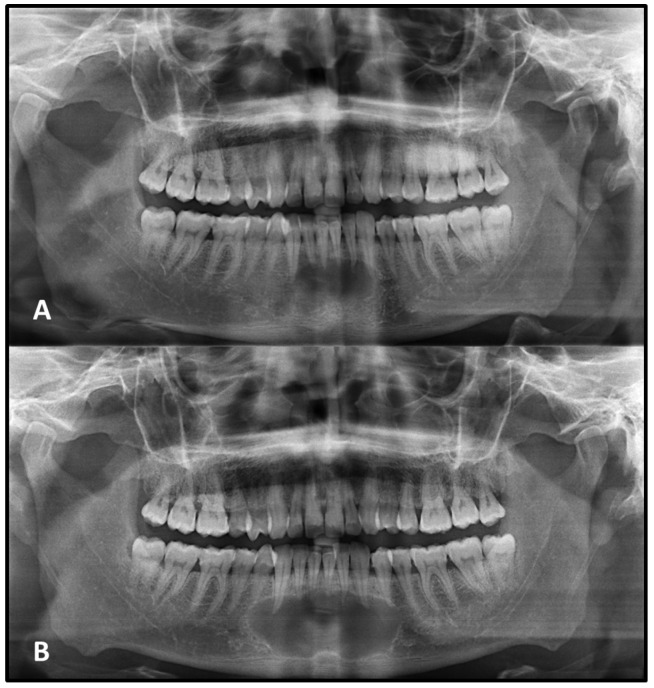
A radicular cyst located in the anterior mandible with well-defined but non-corticated borders is seen on the initial panoramic radiograph (A). After two and a half years, the lesion showed considerable enlargement and developed continuous corticated margins (B).
